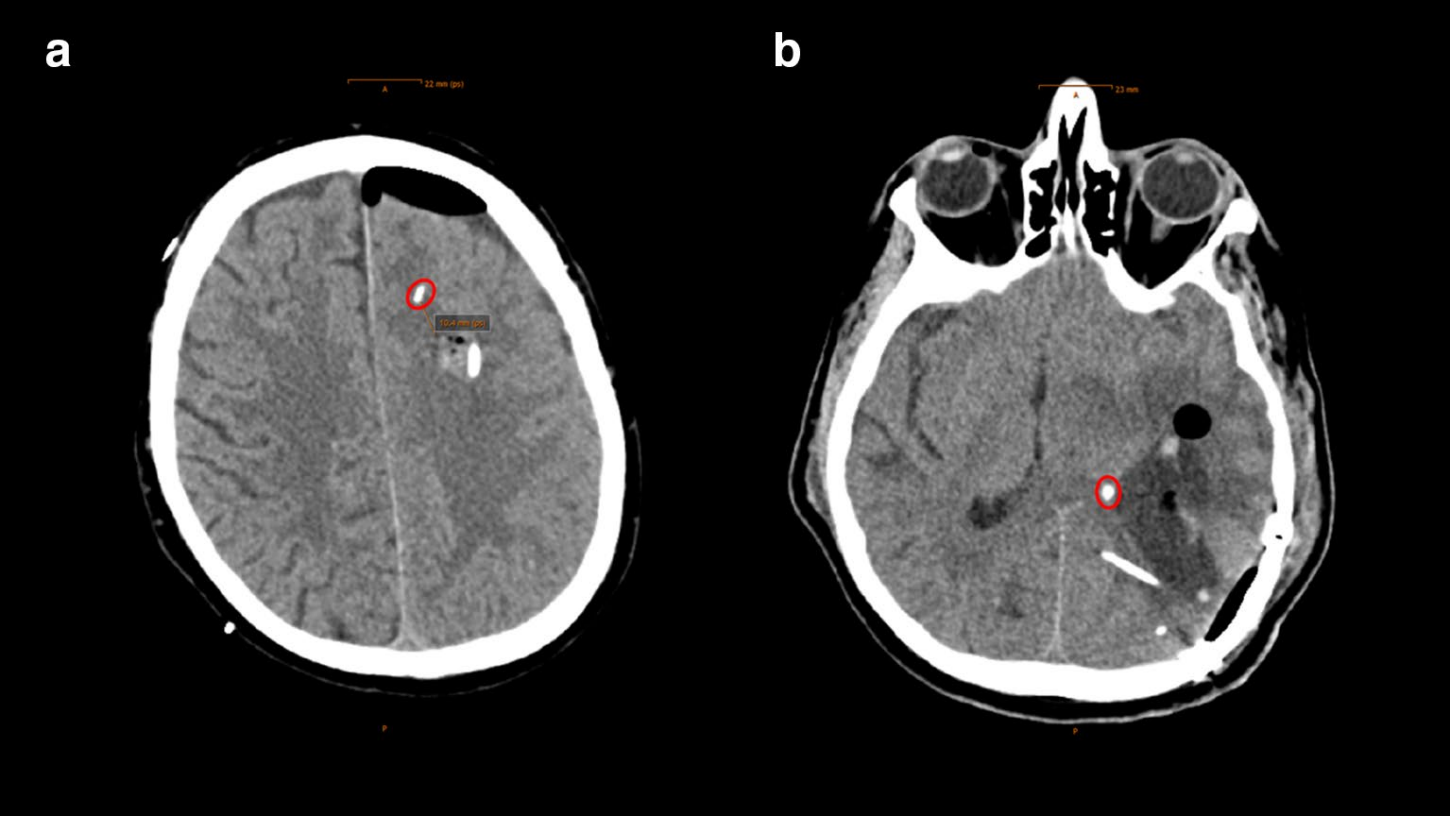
Figure shows examples for probe location in healthy brain tissue (a) and perilesional location (b) on axial computed tomography scans of the brain
